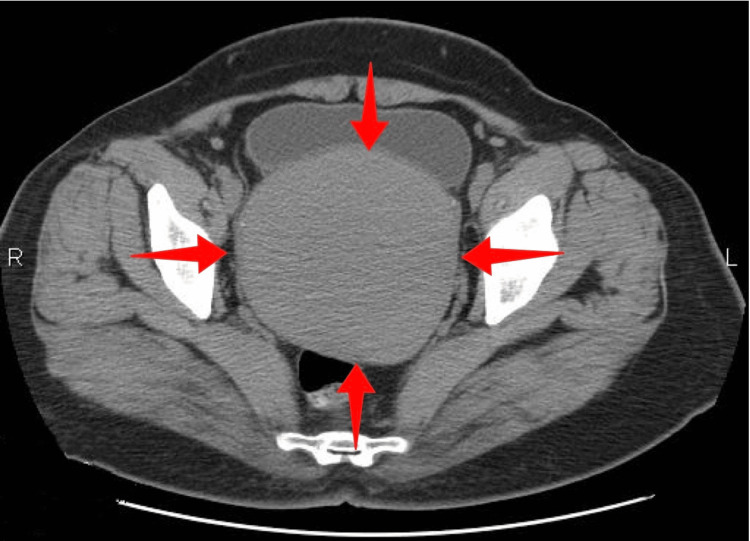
Computed tomography imaging of uterine cervical leiomyoma (our case) The pelvic mass depicted within the red arrows corresponds to a large cervical leiomyoma.
